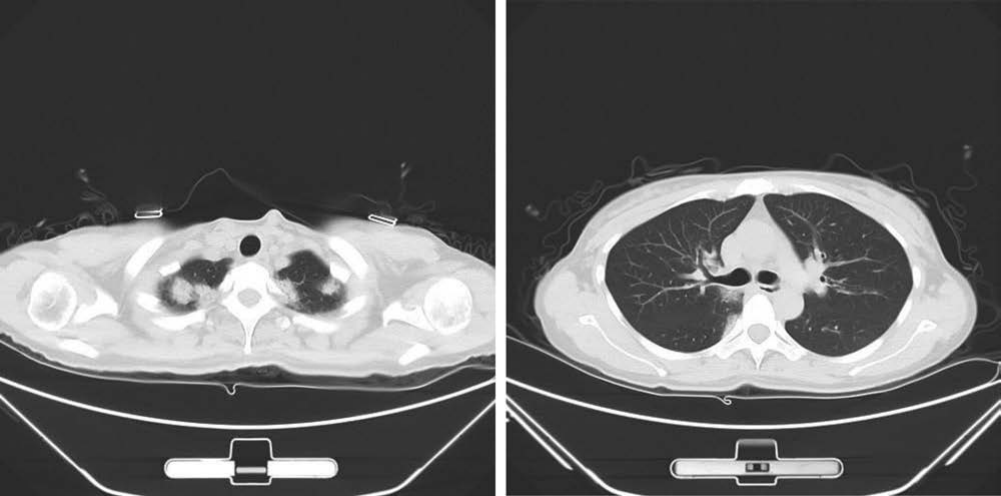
CT of the chest revealed multiple patchy opacities in both lungs.
